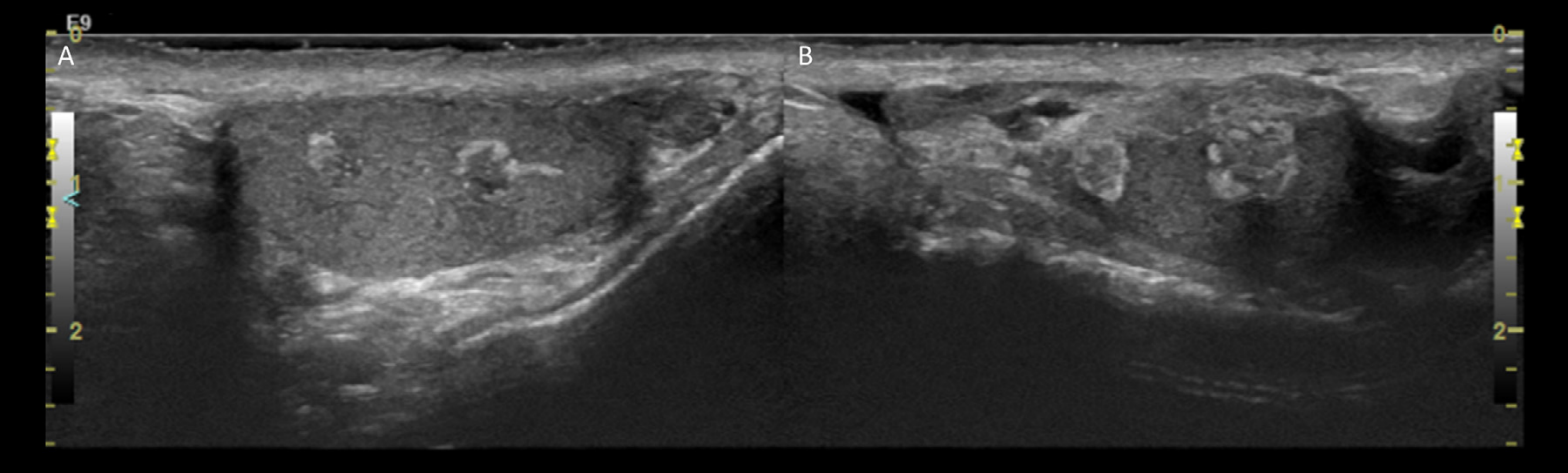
Transverse ultrasound of the bilateral testes reveals normal testicular size with numerous mixed echogenicity masses in the mediastinum testis ranging in size from 3 to 6 mm. Imaging features and clinical presentation were compatible with testicular adrenal rest tumors.
